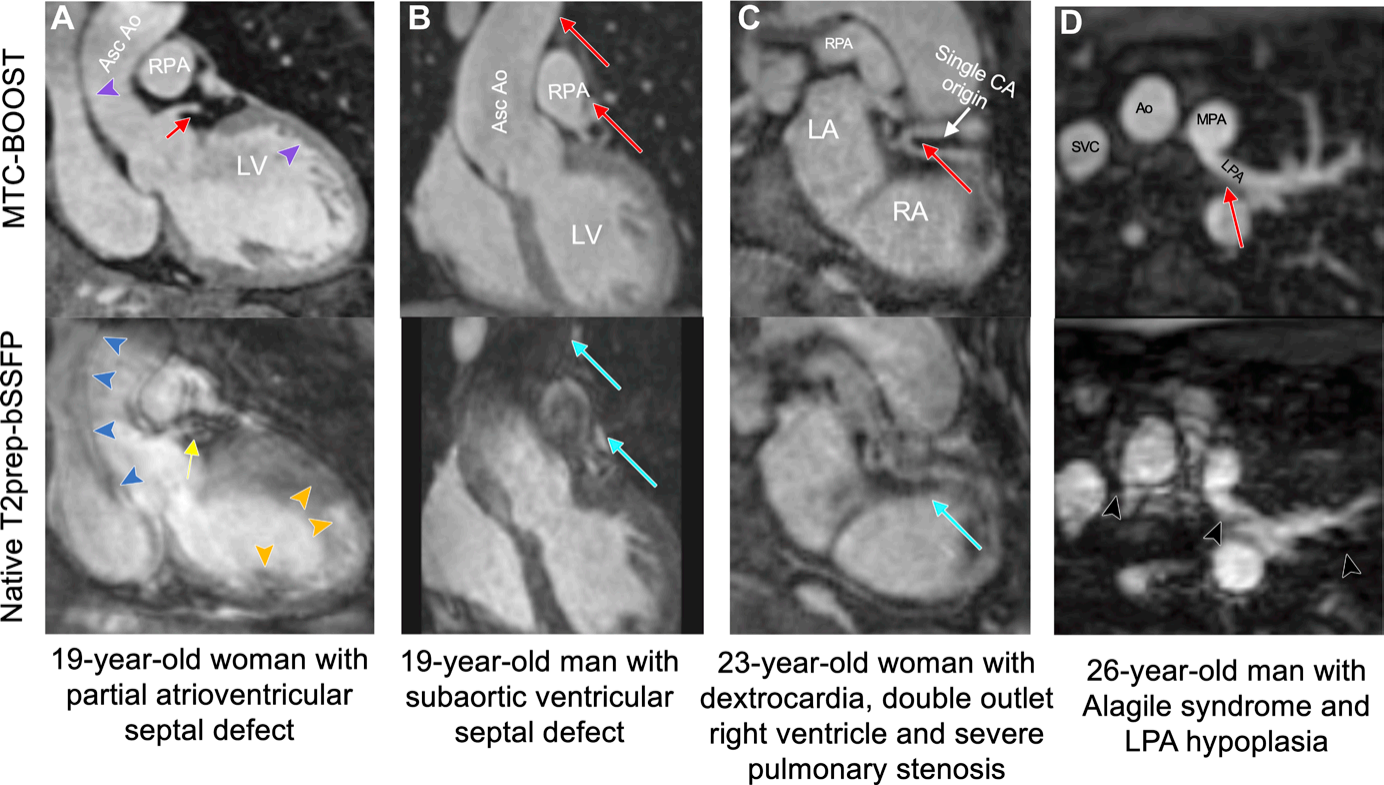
Comparison of MTC-BOOST and native T2prep-bSSFP cardiac MRI.
